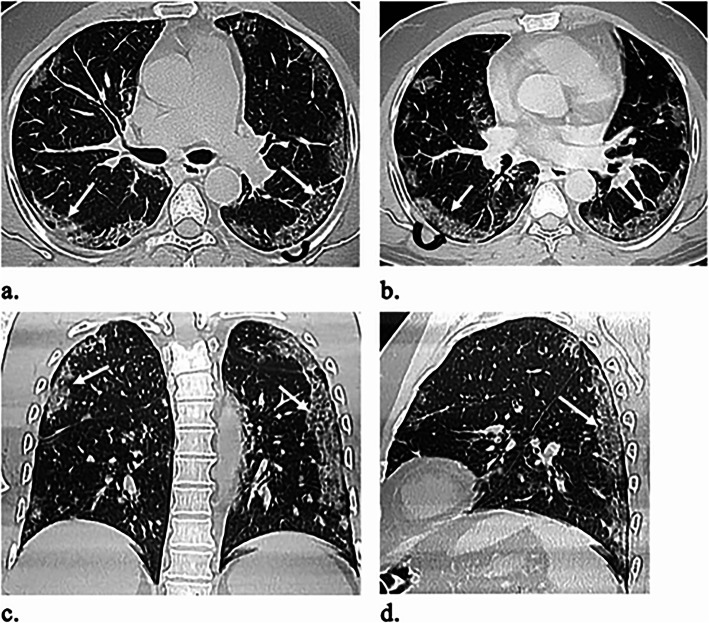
A 54-year-old male patient presented with 8 days history of breathlessness and cough. O_2_ saturation was 93%. a, b Axial, c coronal, and d sagittal unenhanced HRCT images show bilateral multifocal, mainly subpleural infiltrations with GGOs and crazy paving (white arrows). Subpleural sparing is noted (curved black arrows)
